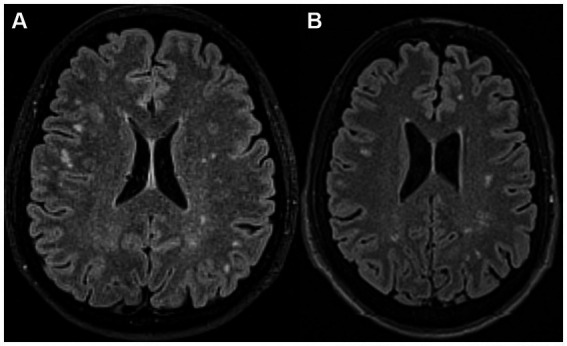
(A) A 46-year-old female patient with migraine with aura. The axial fluid-attenuated inversion recovery (FLAIR) MR image shows multiple white matter lesions (WMLs) in both cerebral hemispheres. (B) A 32-year-old man with relapsing–remitting multiple sclerosis. The axial FLAIR MR image shows multiple WMLs in both cerebral hemispheres.
